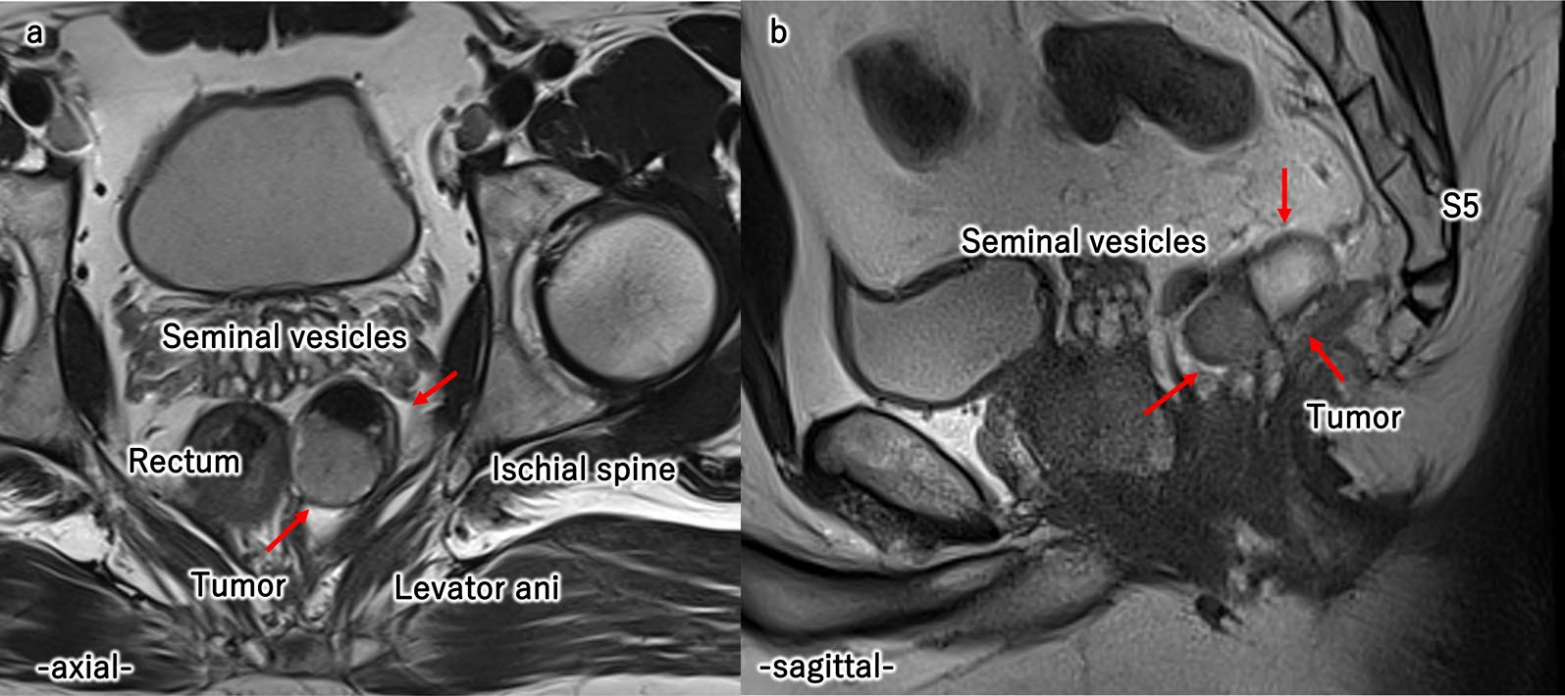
Abdominal MRI. The tumor contained highly and iso-dense areas. Enhanced magnetic resonance imaging (MRI) showed a multilocular cystic tumor with high and low signal intensity on T2-weighted images (a, b). The tumor had no contrast effect
